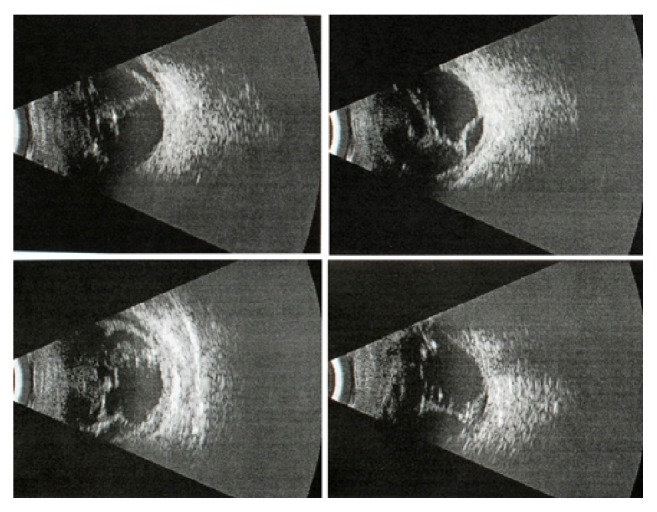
Retinal detachment seen in ultrasonography in patient with corneal laceration.
